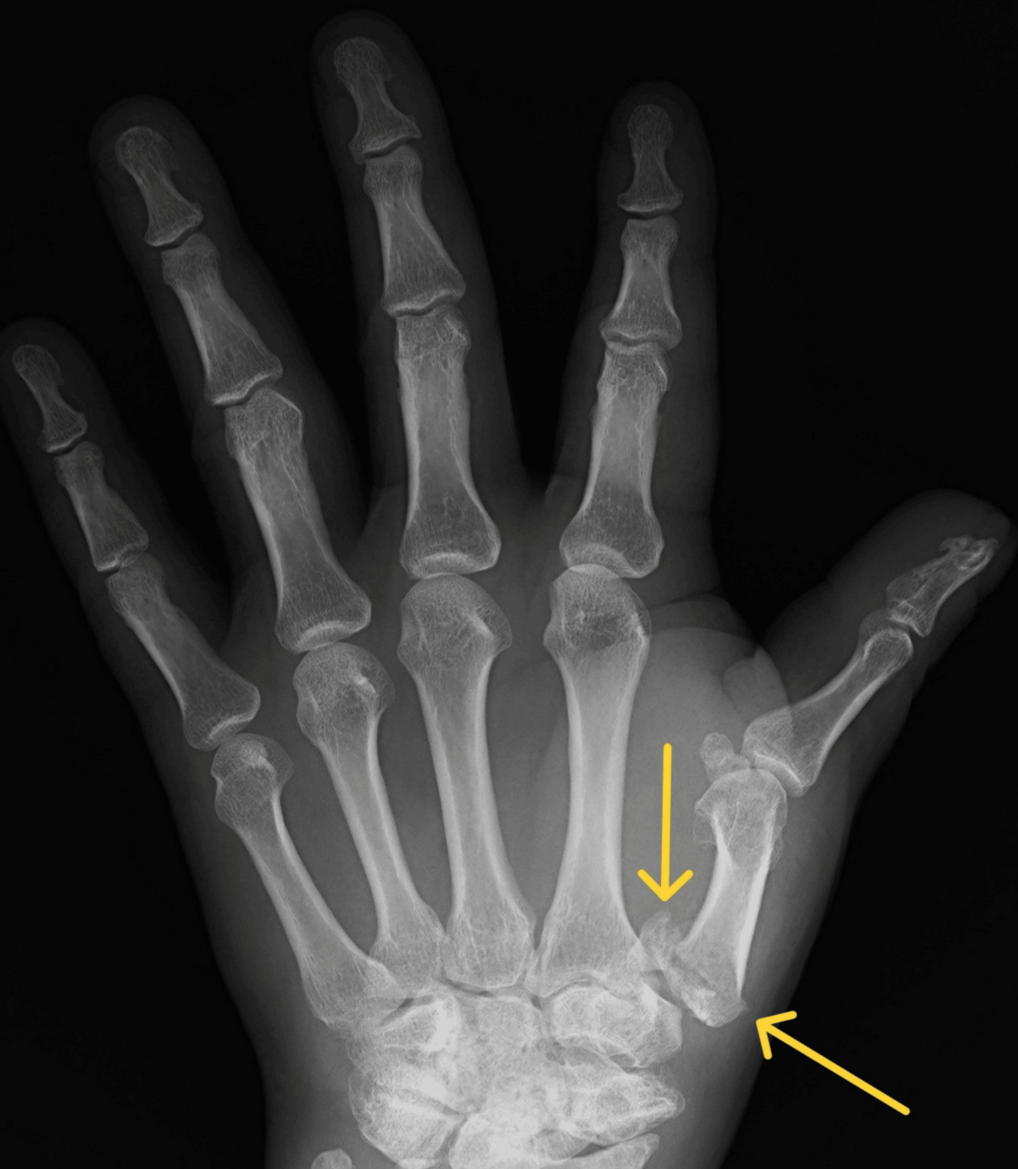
Rolando fracture with subluxation of the CMC joint of the first metacarpal bone (X-ray in A-P projection) CMC: carpometacarpal; A-P: anterior-posterior
